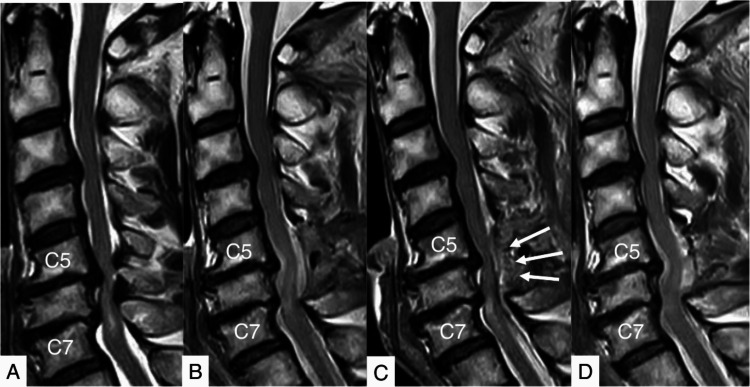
Pre- and postoperative magnetic resonance images (MRI) of Case 3. A: A preoperative sagittal MRI shows severe cord compression at C5/6 and C6/7. B: A postoperative sagittal MRI shows posterior decompression from C5 to C7. C: An extradural hematoma compressing the spinal cord (white arrows) was identified four days after surgery. D: The postoperative extradural hematoma was resolved after hematoma evacuation.
